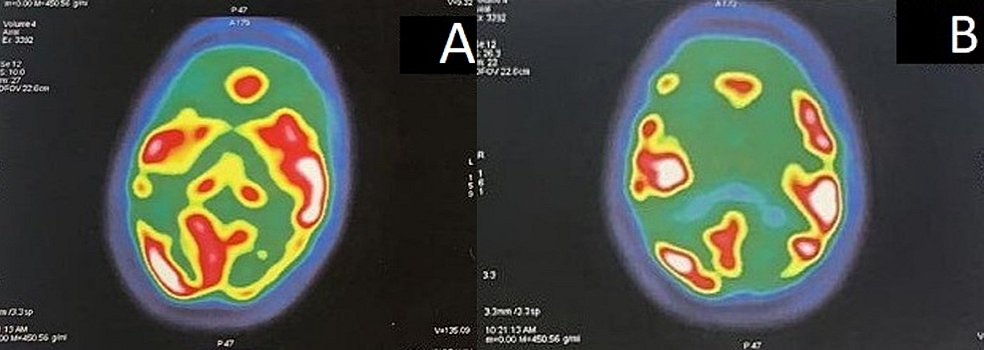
[18F] fluoro-2-deoxy-D-glucose positron emission tomography (FDG-PET); FDG-PET reduced glucose metabolism.
